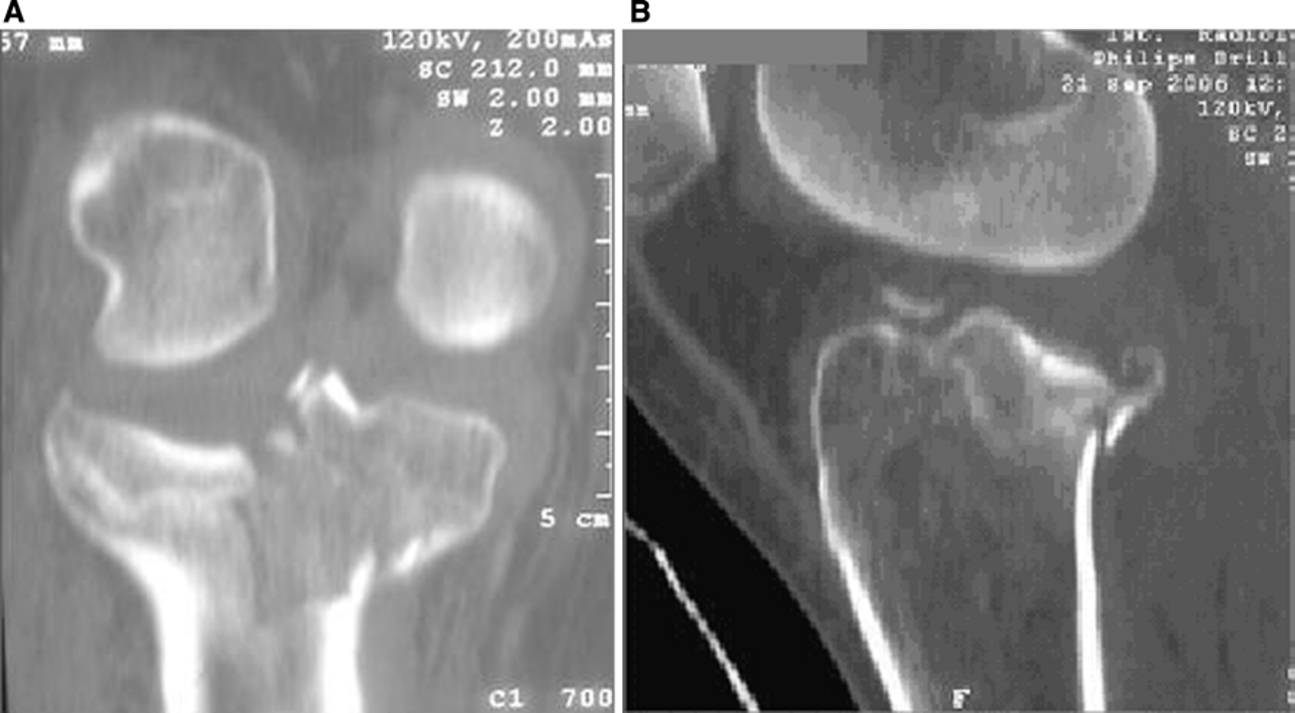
a Schatzker type V fracture, CT scan. b Schatzker type V fracture, CT scan
